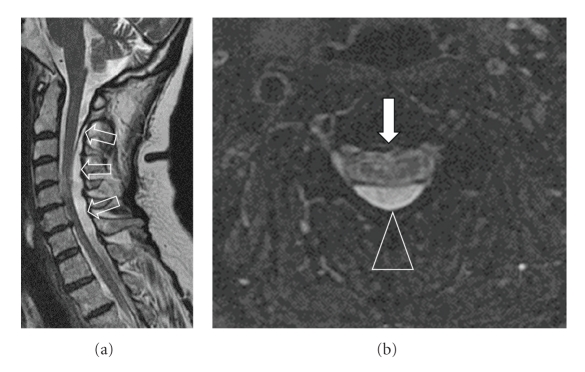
MRI findings at reassessment. Cystic cavity extending from cervicocranial junction to the T4 vertebral body with myelon displacement ((a)-*open arrows*). A thick membrane separated the T2-hyperintense mass ((b)-*triangle*) from the spinal cord ((a)-*long arrow*). Note myelopathy signal at vertebral body heights C4/5 and disc heights C6/7 on T2-weighted (a) and T2*-weighted images ((b)-*filled arrow*).
